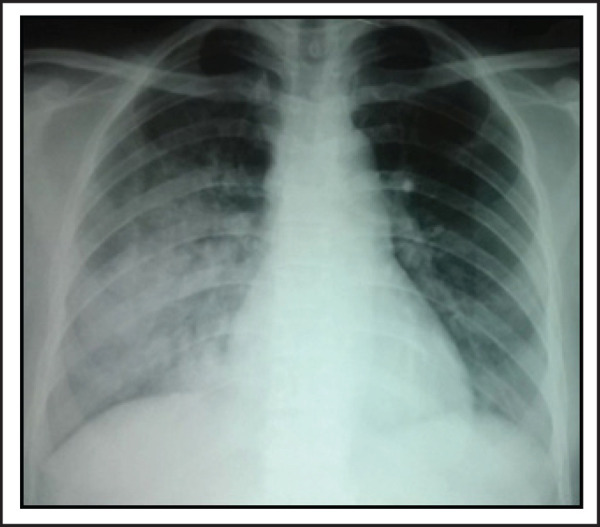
Chest X-ray showing bilateral alveolar opacities in the lower and middle zones.
